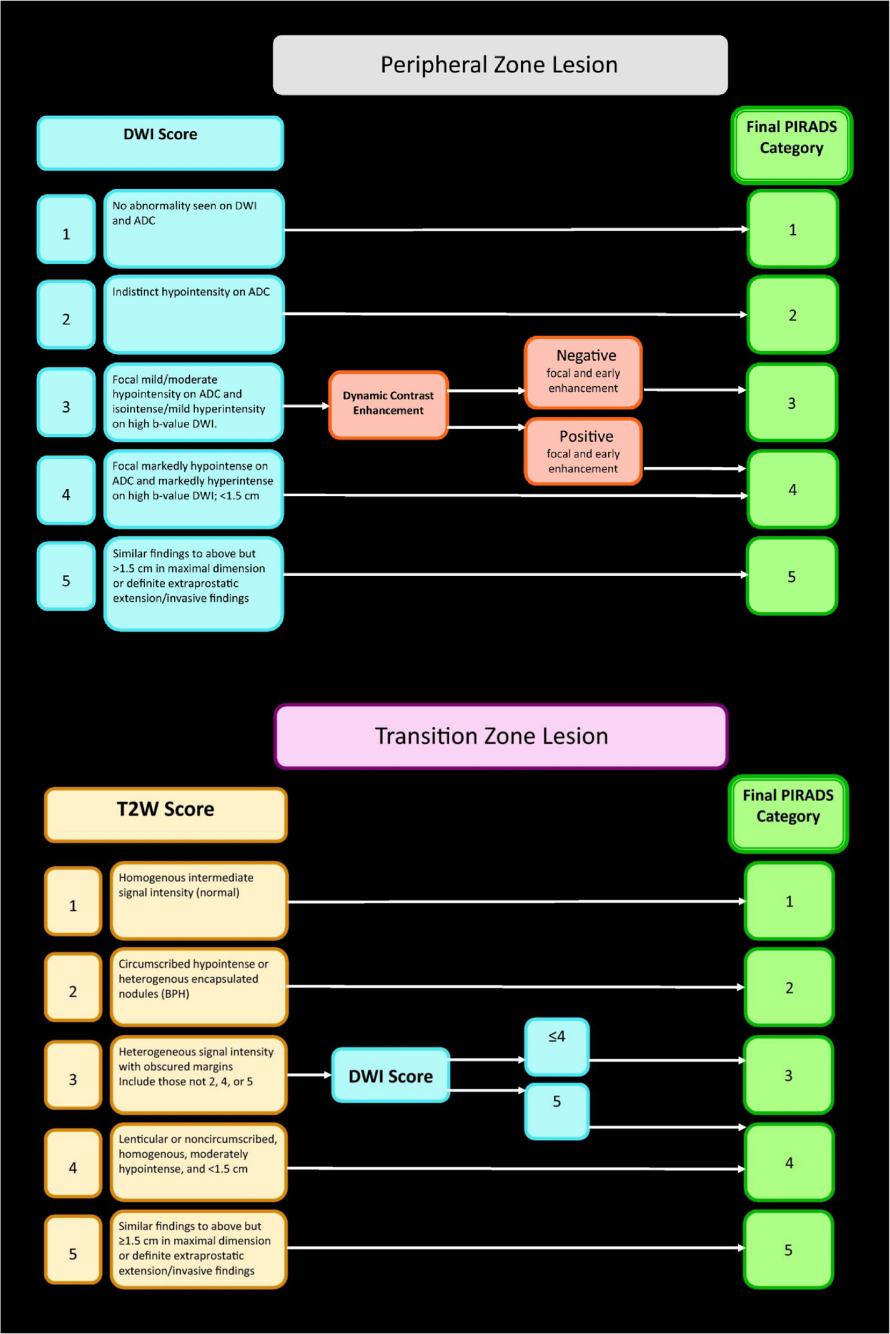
The predominant sequence is DWI in the peripheral zone and T2w sequence in the transition zone. In case of PI-RADS assessment category 3 in the peripheral zone DCE-MRI determines the final PI-RADS assessment category and in PI-RADS assessment category 3 in the transition zone DWI determines the final PI-RADS assessment category. When image quality is compromised and DCE-MRI is inadequate for the assessment of the peripheral zone in PI-RADS assessment category 3 the PI-RADS score is defined by DWI alone. If DWI is not adequate PI-RADS™ v2 guidelines advice use of T2w sequence for both, the peripheral zone and for the transition zone. If both DWI and DCE-MRI are inadequate or not available, assessment should be limited to staging for determination of EPE [11]
